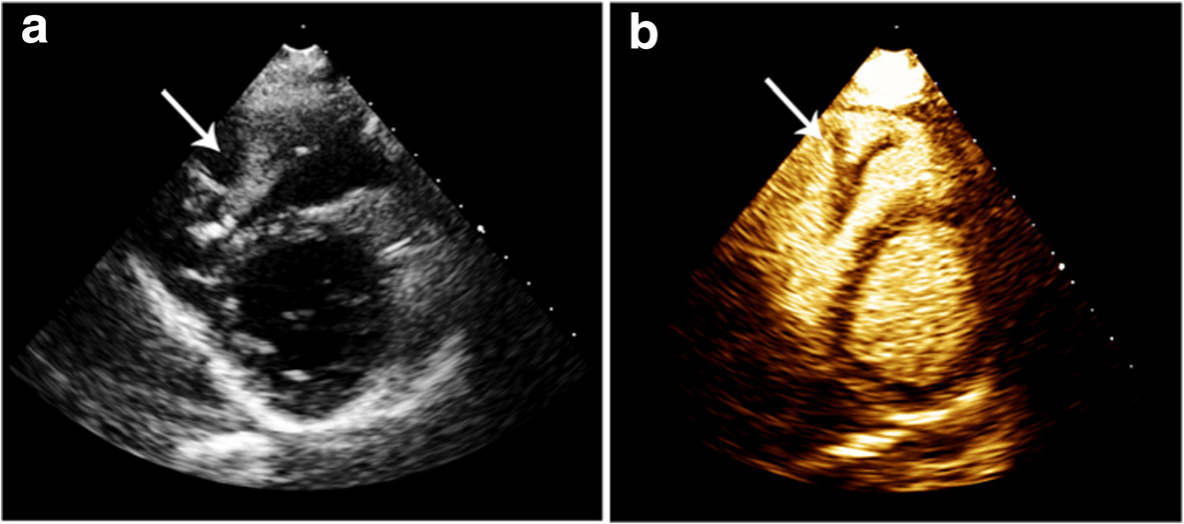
Transthoracic echocardiography showing a giant right intra-ventricular structure (arrow) in a parasternal short axis view (Panel a). The characteristic lack of contrast enhancement in the right intra-ventricular cavity confirms the presence of a giant right ventricular thrombus (Panel b)
